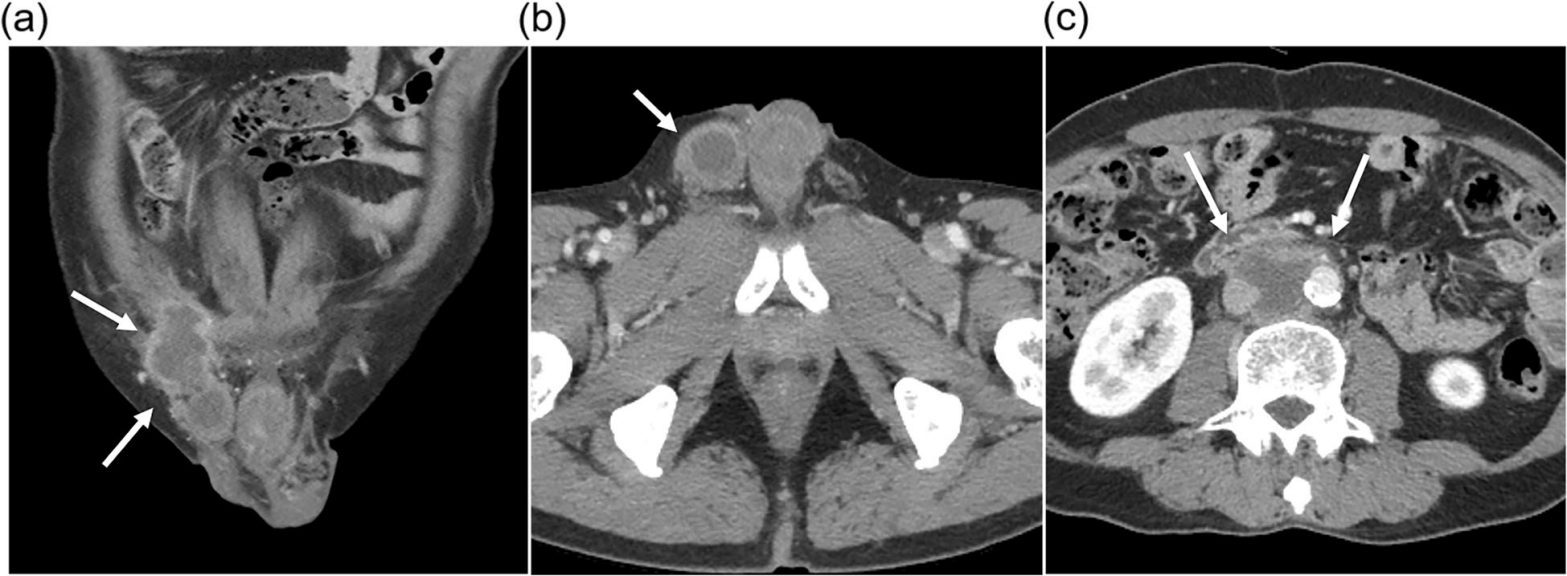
Computed tomography images. a An irregular elongated mass (arrows) along the right inguinal canal and right external iliac vessels (b) A round mass (arrow) at the lower portion of right inguinal canal, probably arising from the right testis. c Another mass (arrows) at the aortocaval space at the L3 level indicating metastatic lymphadenopathy
